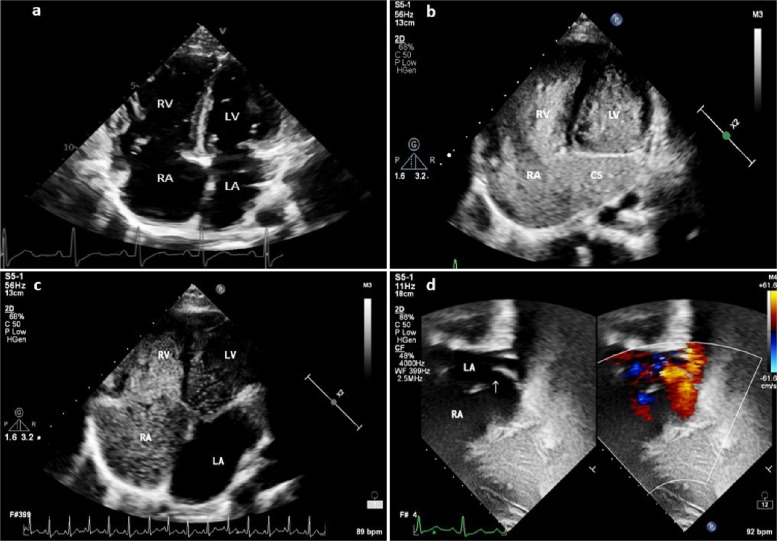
Apical four-chamber views and subcostal sagittal view. A Apical four-chamber view demonstrating dilated right atrium (RA) and right ventricle (RV). B Apical four-chamber view after contrast injection through left antecubital vein demonstrating the appearance of contrast bubbles in coronary sinus (CS), left ventricle (LV), RA, and RV. C Apical four-chamber view demonstrating the appearance of contrast bubbles in LV, RA, and RV with no apparent contrast opacification in left atrium (LA). D Subcostal sagittal view demonstrating dilated CS and defect in the roof of CS (arrow). Color flow doppler (color comparison on the right) shows a red jet from LA to CS to RA
